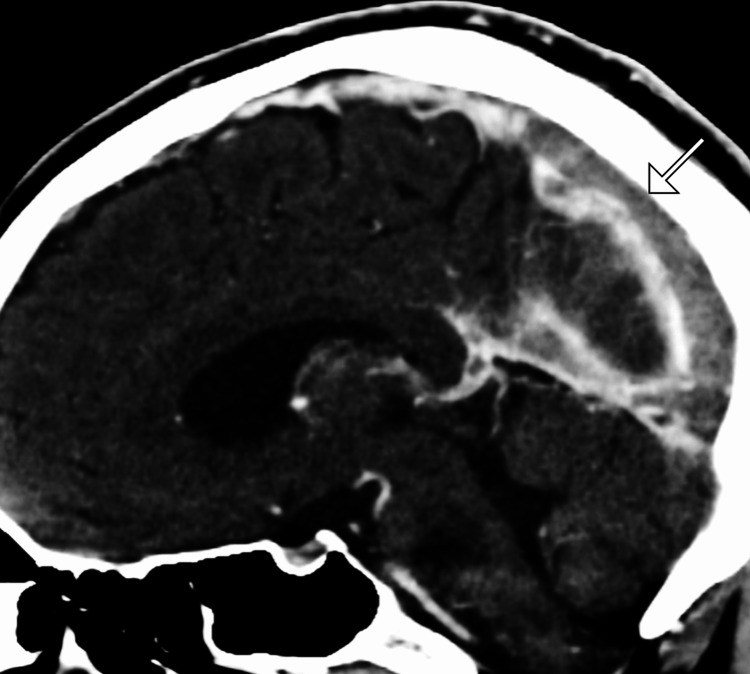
Sagittal CTV shows filling defect along the superior sagittal sinus (arrow) CTV: computed tomography venography
